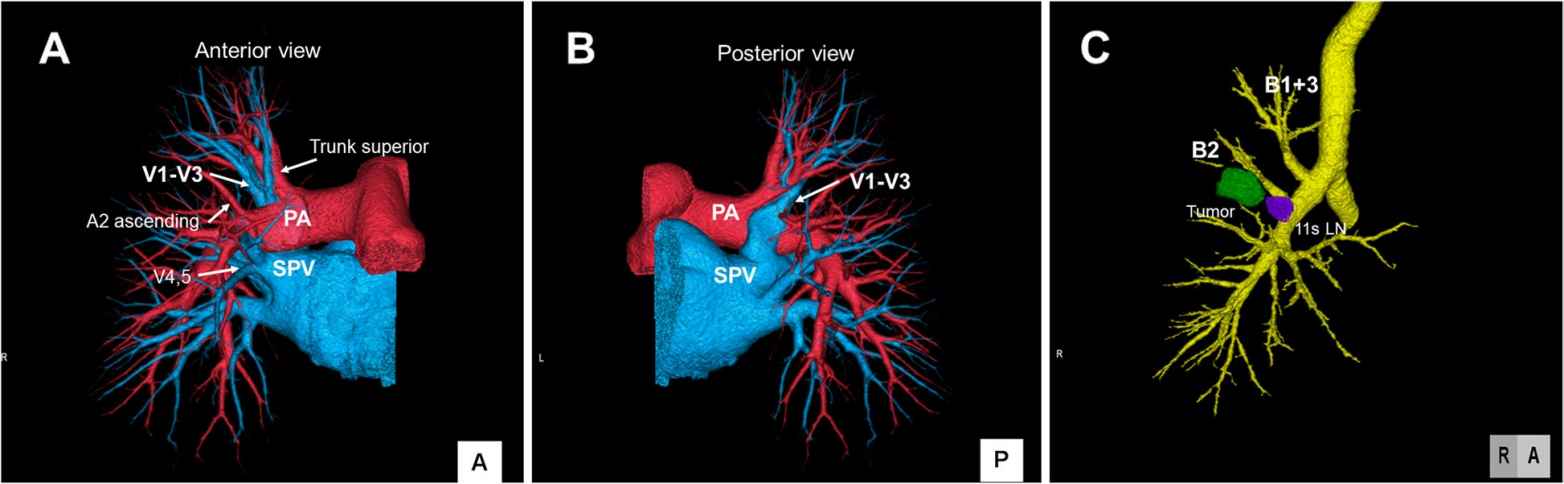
A A 3D-CTA scan (anterior view) showing the V1–V3 segment passing dorsal to the right main PA. B A 3D-CTA scan (posterior view) showing the V1–V3 segment draining into the SPV. C The B2 and B1 + 3 segments are observed to branch separately from the right main bronchus. The tumors are marked green and the station 11s lymph nodes appear purple. LN: lymph node, PA: pulmonary artery, SPV: superior pulmonary vein, 3D-CTA: three-dimensional computed tomography angiography
